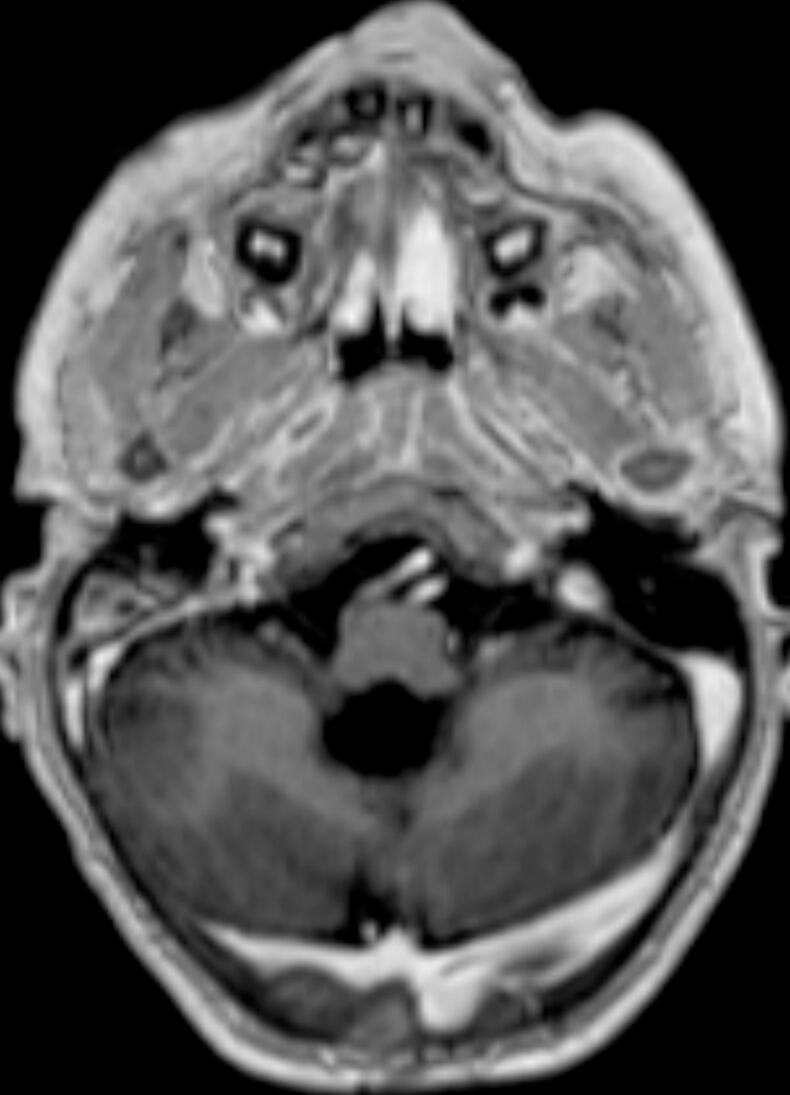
Axial cut of a T1 MRI seqquence done on Febraury 2025. There is no evidence of radiological.
